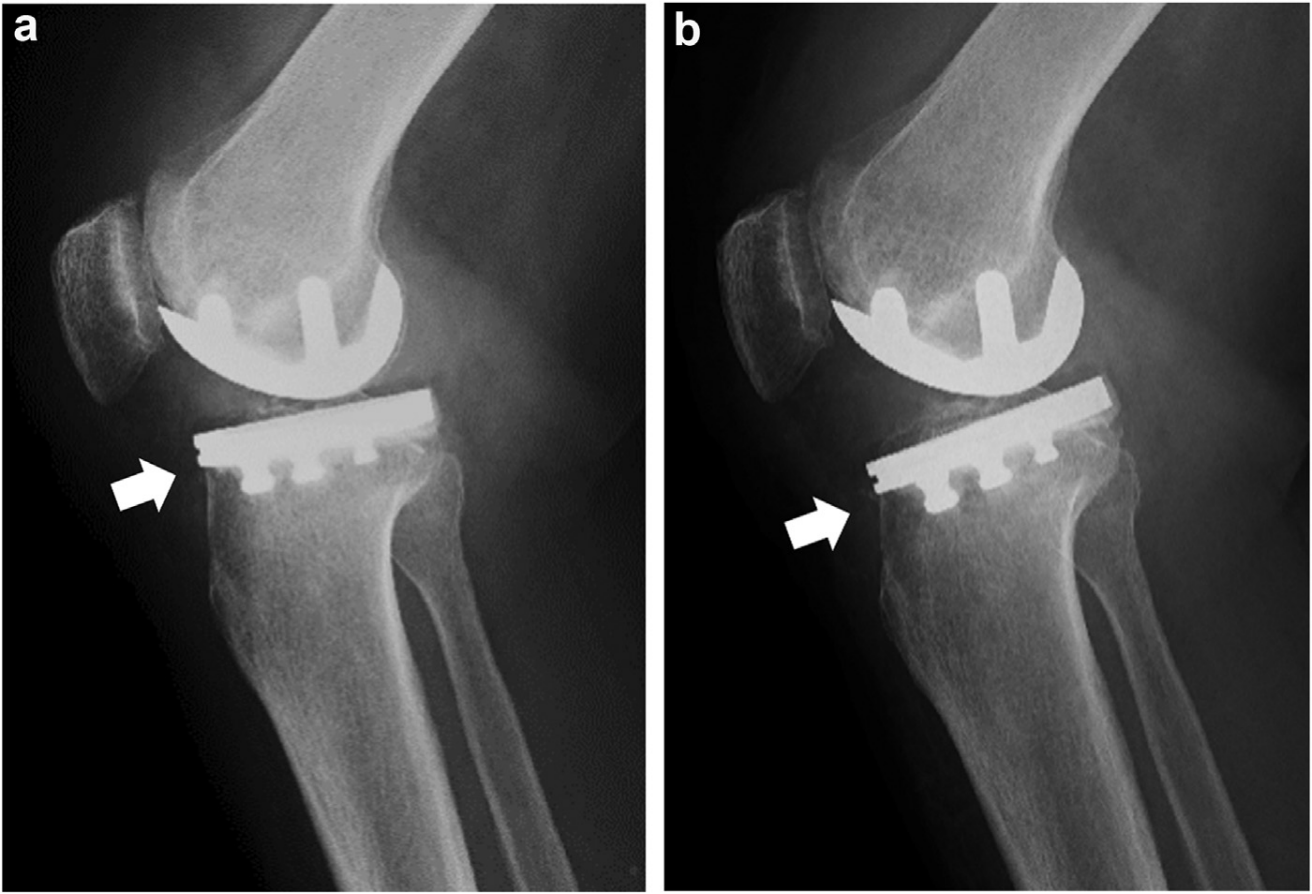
Case 4, 70-year-old female. (a) Postoperative radiography. Anterior tibial cortical support was achieved (arrow). (b) Three months after surgery, anterior collapse of the tibial component was observed (arrow).
